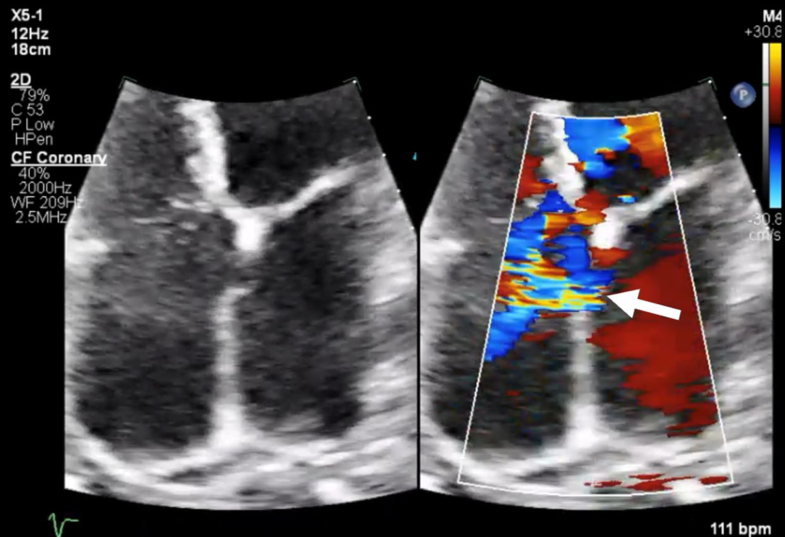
Transthoracic Echocardiogram 4-Chamber View Showing Left-to-Right Atrial Shunt by Color Doppler (Arrow)
